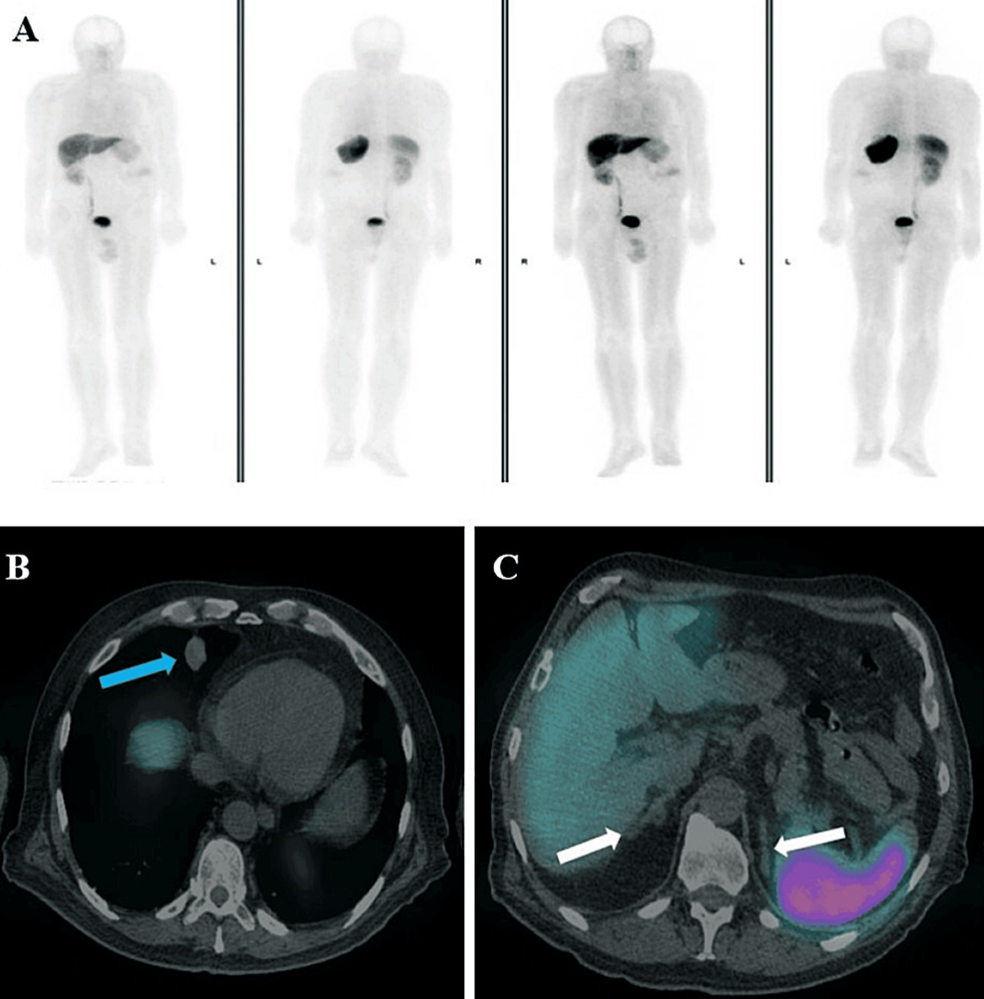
Octreotide single photon emission computed tomography and computed tomogram (SPECT CT) whole body maximum intensity projection (MIP) with no significant uptake (A) and planar trans-axial CT fusion images at 30-minute delay showing no significant tracer uptake in relation to the right middle lobe pulmonary nodule (blue arrow) and adrenal glands (white arrows) (B, C).
